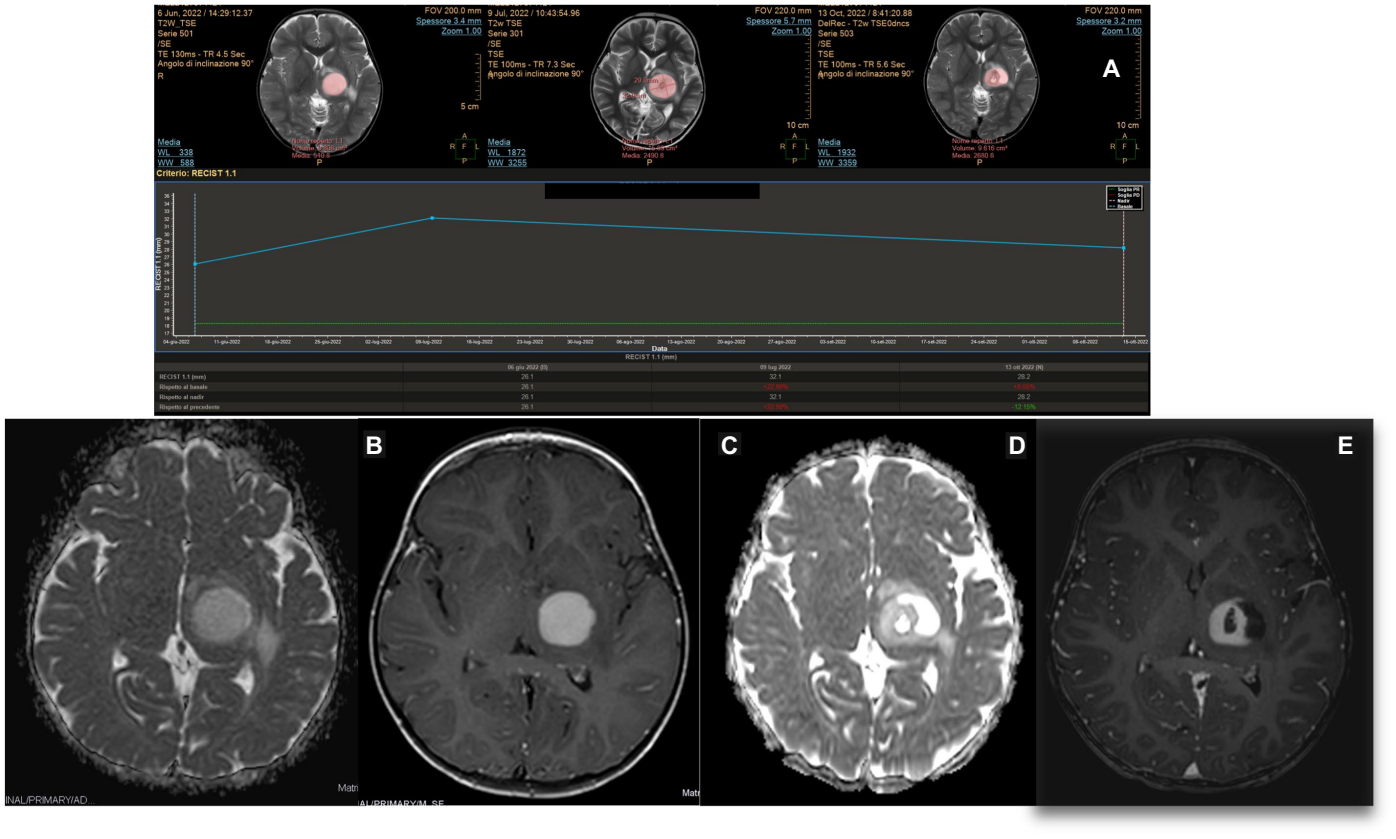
(A) MITT Intellispace Portal imaging examination. After 1 month of laser interstitial thermal therapy, when chemotherapy had not yet started, the tumor on diffusion (d) was 22% bigger because of intralesional edema. At 4 months, it was reduced by an overall 22%. (B,C) Pre-operative magnetic resonance imaging (MRI) on ADC and T1 TFE 3D post-enhancement imaging. (D,E) ADC and post-contrast-enhancement MRI obtained 5 months after surgery and after four cycles of chemotherapy demonstrated a cystic evolution of the tumor, with a clear reduction of the contrast enhancement and the involution of the central necrotic part.
